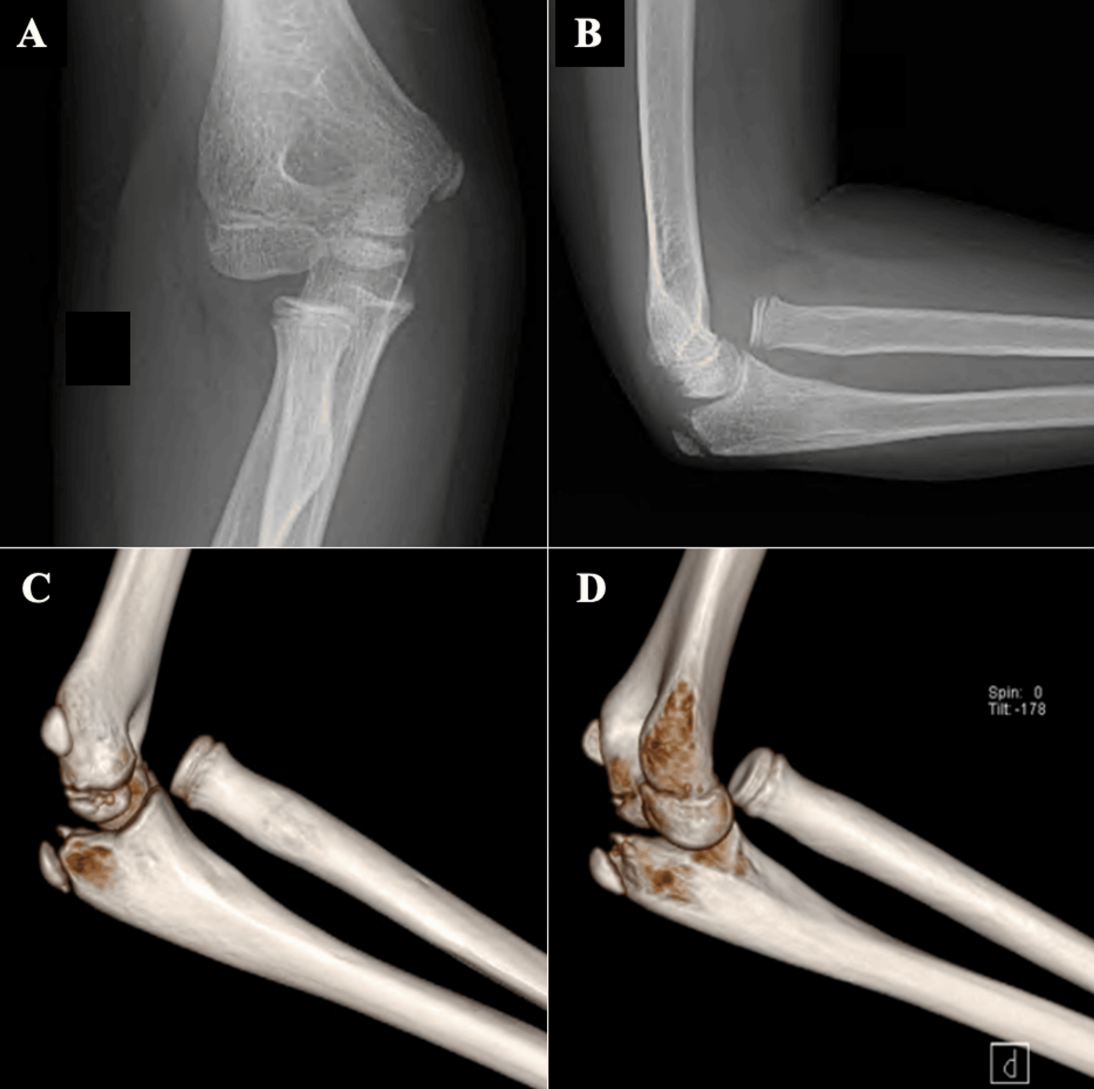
X-ray and computed tomography scan of radial head dislocation of the right elbow. 1A shows an anteroposterior radiographic view of radial head dislocation of the right elbow; 1B shows a lateral radiographic view of radial head dislocation of the right elbow; 1C and 1D show a 3D reconstruction of a computed tomography scan of radial head dislocation of the right elbow.
